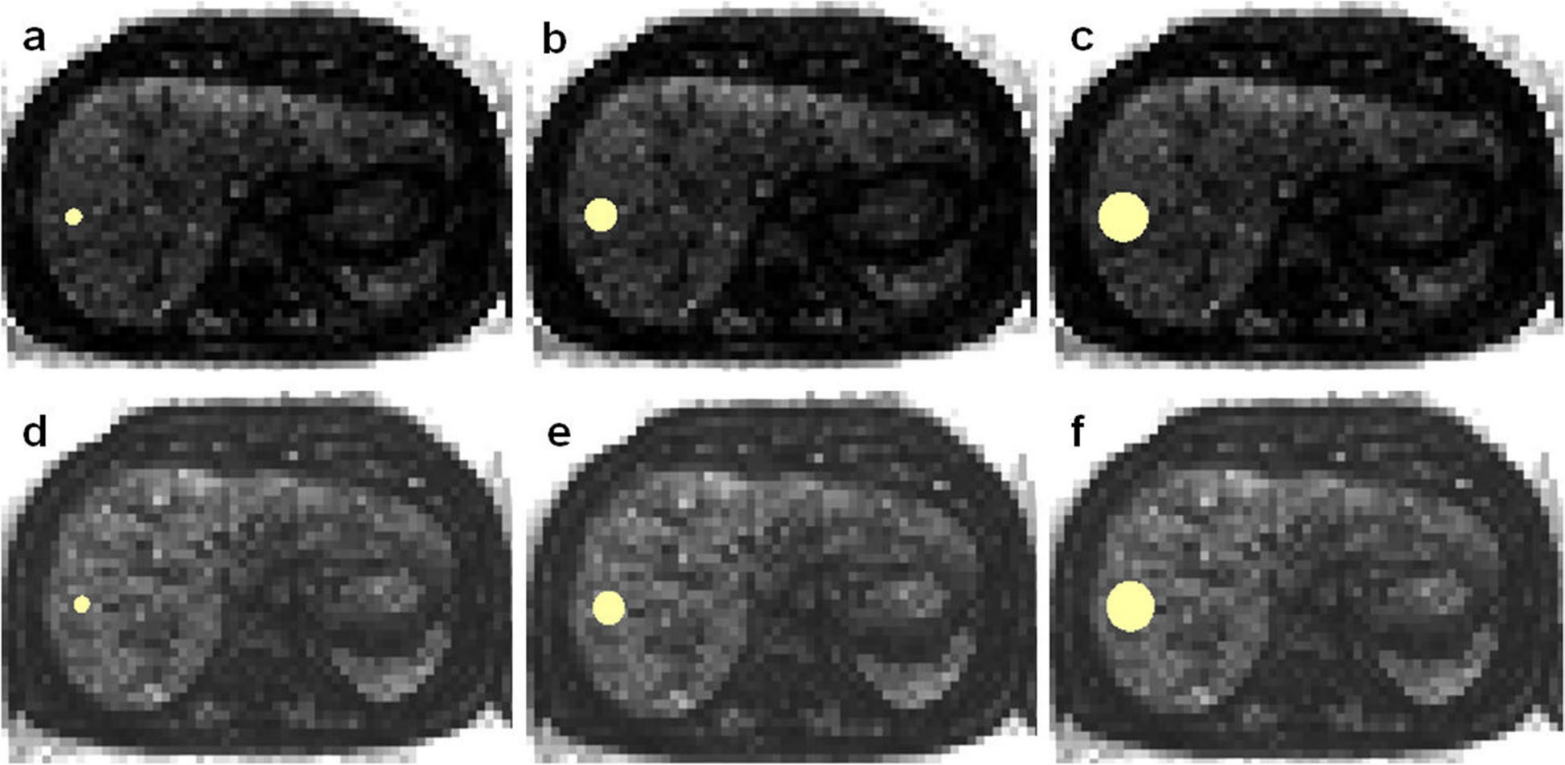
Volume of interest copy in the feature maps. Map of the feature first-order uniformity of the same patient as shown in Fig. 1. a–c Maps derived from T2-weighted images and (d–f) from the T1-weighted images. The volumes of interest (VOIs) of the conventional extraction were copied to the maps. The mean was extracted from the VOIs, resulting in the feature value for uniformity for the three different VOI sizes
